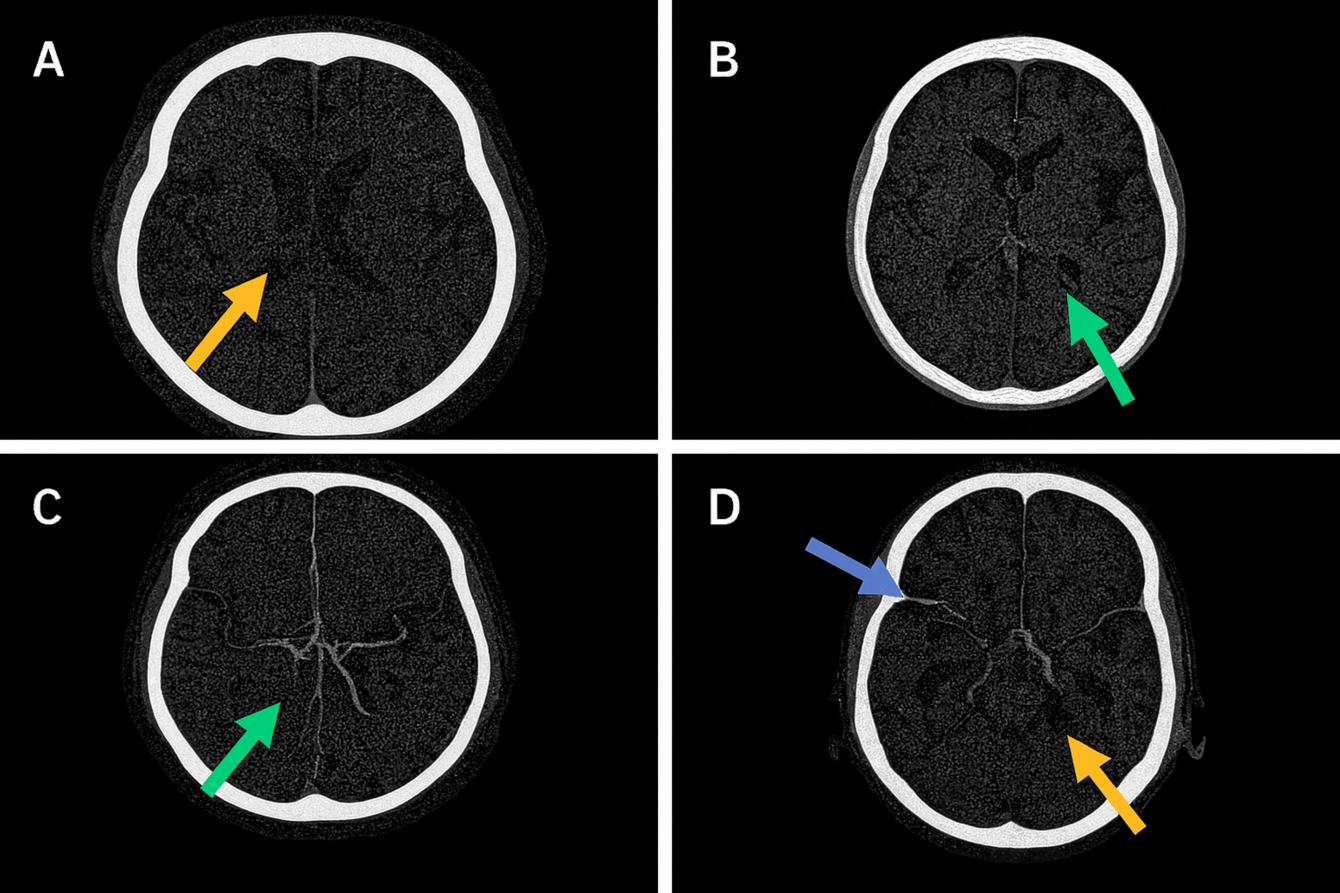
Axial CT angiography images of the brain. A: Normal opacification of the anterior circulation, with symmetric filling of the distal internal carotid arteries and proximal middle cerebral arteries (yellow arrow). No arterial stenosis or occlusion is present. B: The middle cerebral artery branches demonstrate normal contrast enhancement without evidence of vascular narrowing or filling defects (green arrow). C: Continued visualization of patent middle cerebral artery branches with preserved caliber and branching pattern (green arrow). D: Normal opacification of the posterior circulation, including the basilar artery and posterior cerebral arteries (yellow arrow). The superficial cortical veins over the convexity also appear normally opacified (blue arrow). No aneurysm, arteriovenous malformation, or venous thrombosis is identified.
